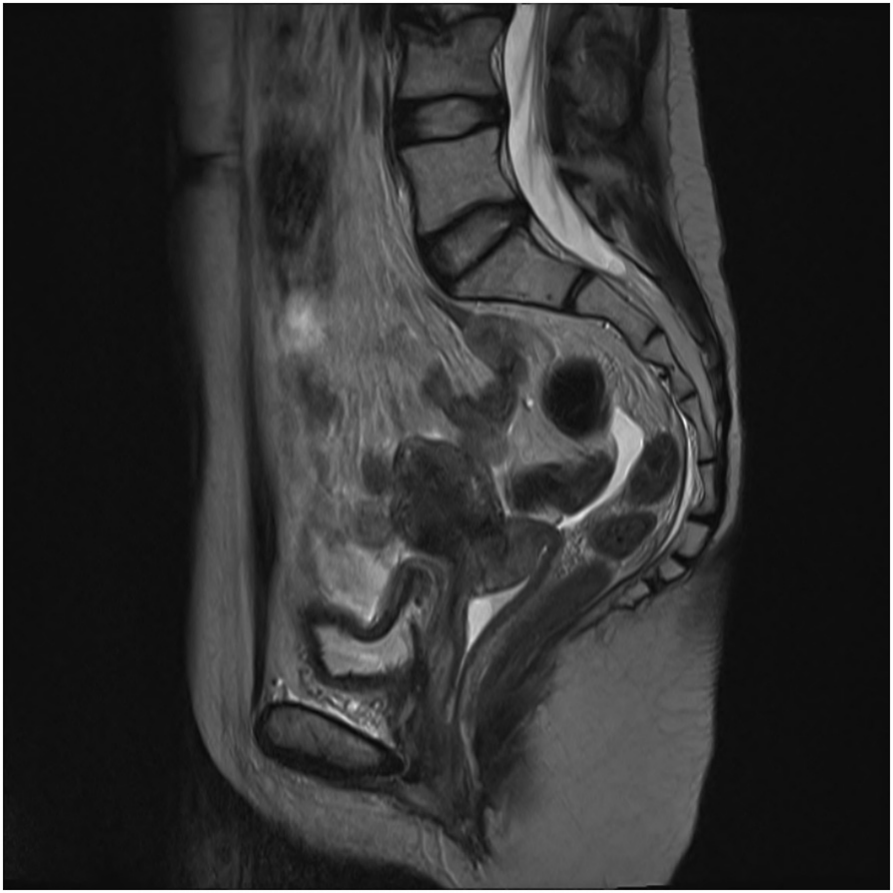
Patient #1: MRI at time of cervical necrosis (January 2009).
